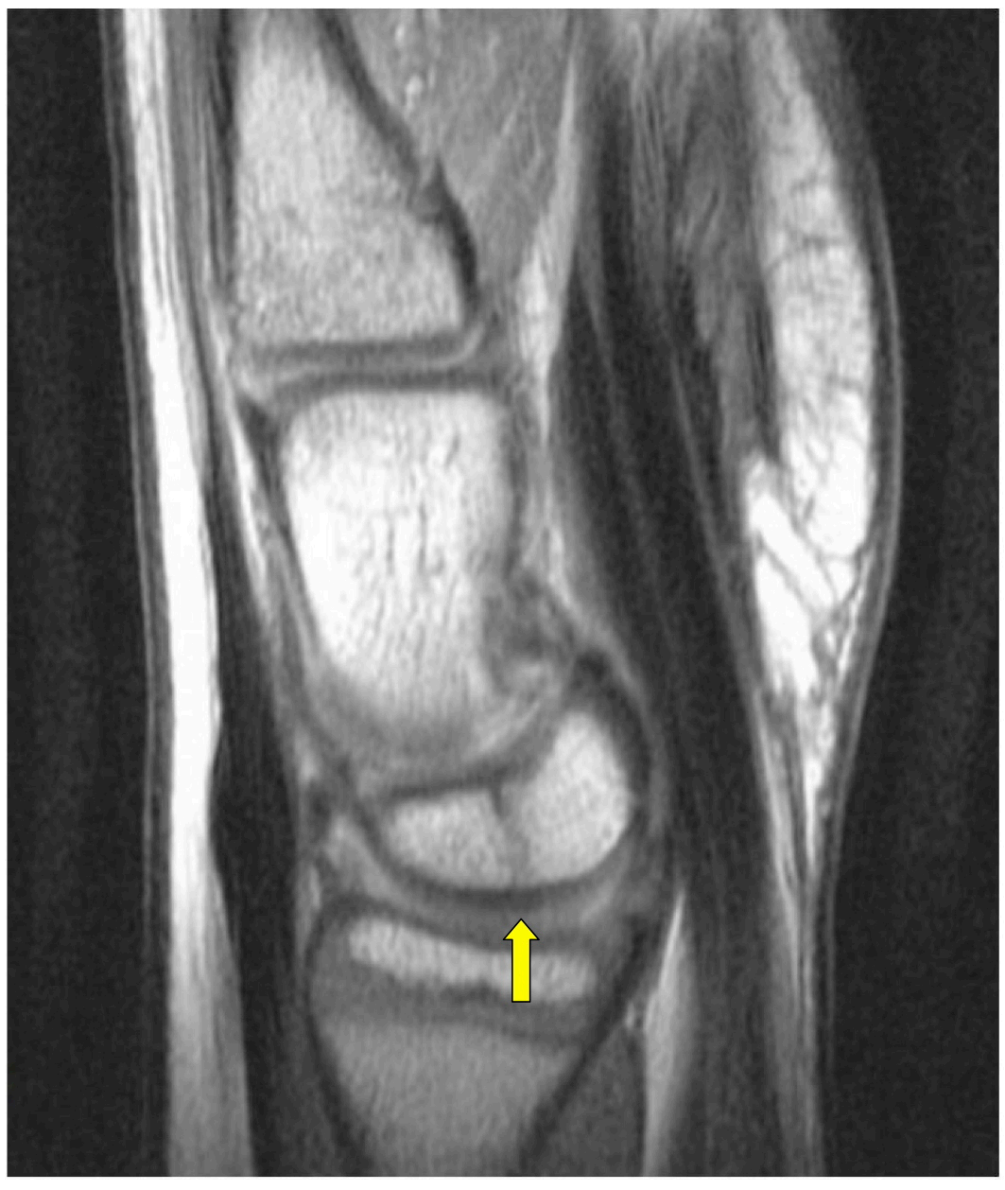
T1-weighted MRI scan of the wrist in the sagittal plane. The divided lunate (arrow) aligns well with the capitate and the radial articular surface.
